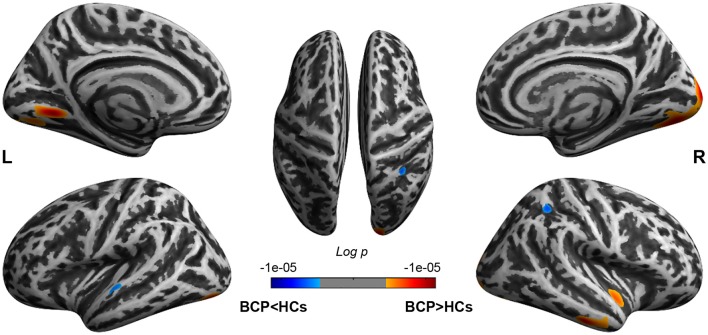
Brain regions show CT differences between BCP and HCs. The thicker cortices are observed in the bilateral hemispheres (lateral occipital, lingual, fusiform), and right hemisphere (cuneus, peri calcarine, inferior temporal, middle temporal, superior temporal, insula). The thinner cortices are observed mainly in the left superior temporal and right superior parietal regions. CT, cortical thickness; BCP, bilateral cerebral palsy; HCs, healthy controls; L, left; R, right.
